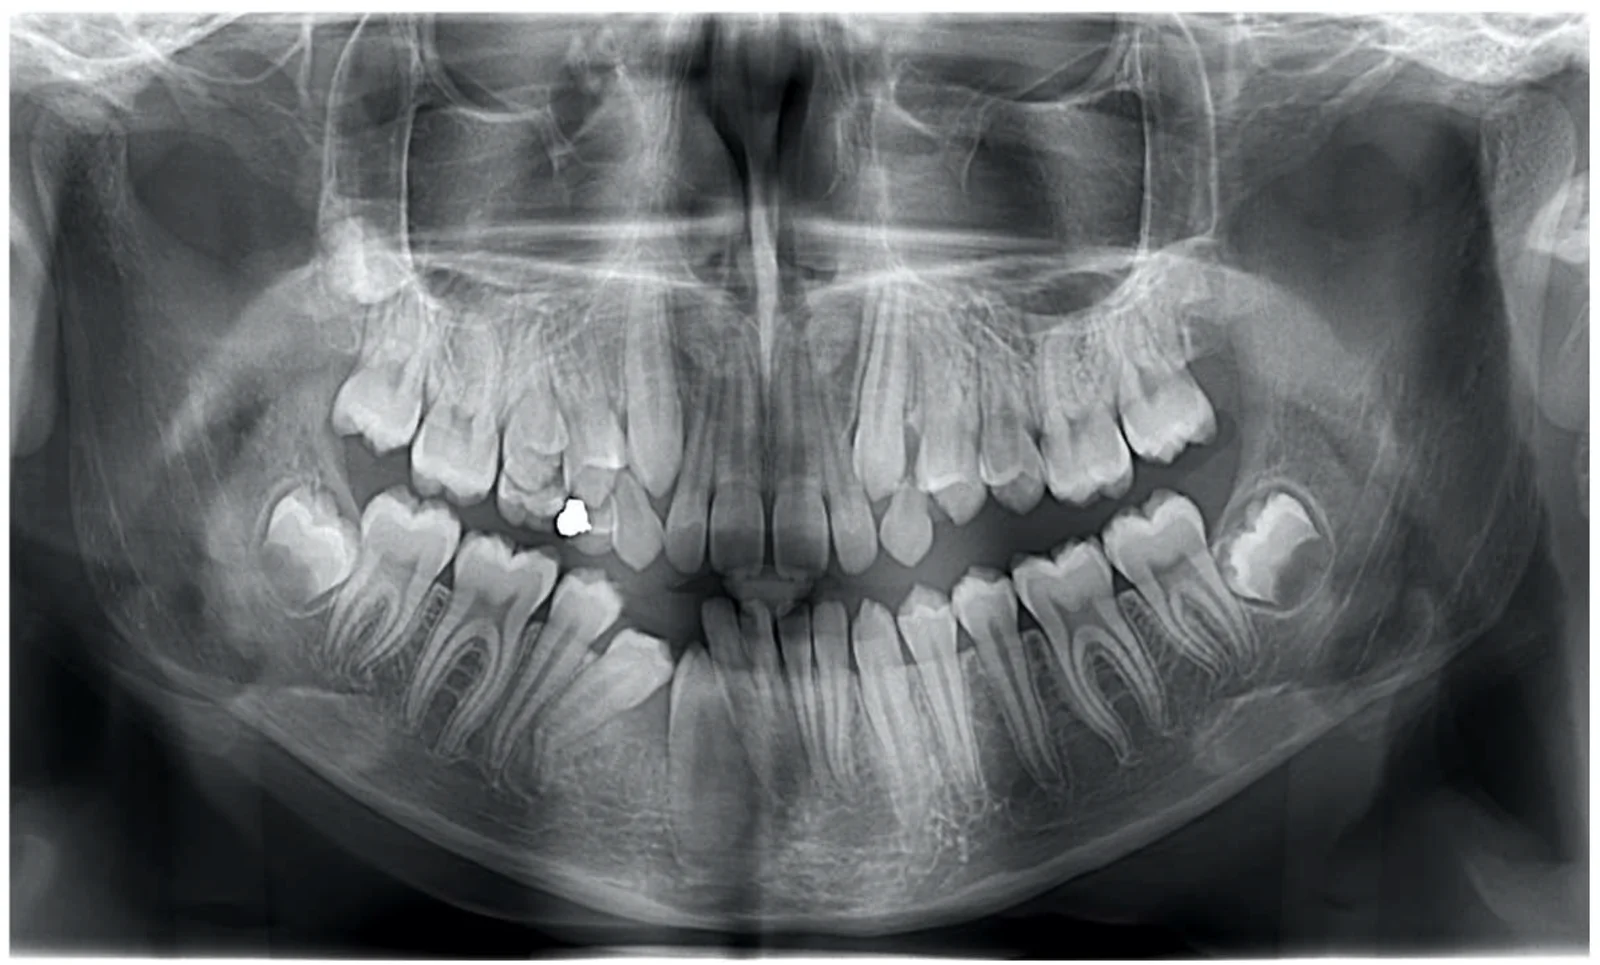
Panoramic radiograph 8.5 months post-surgery.
